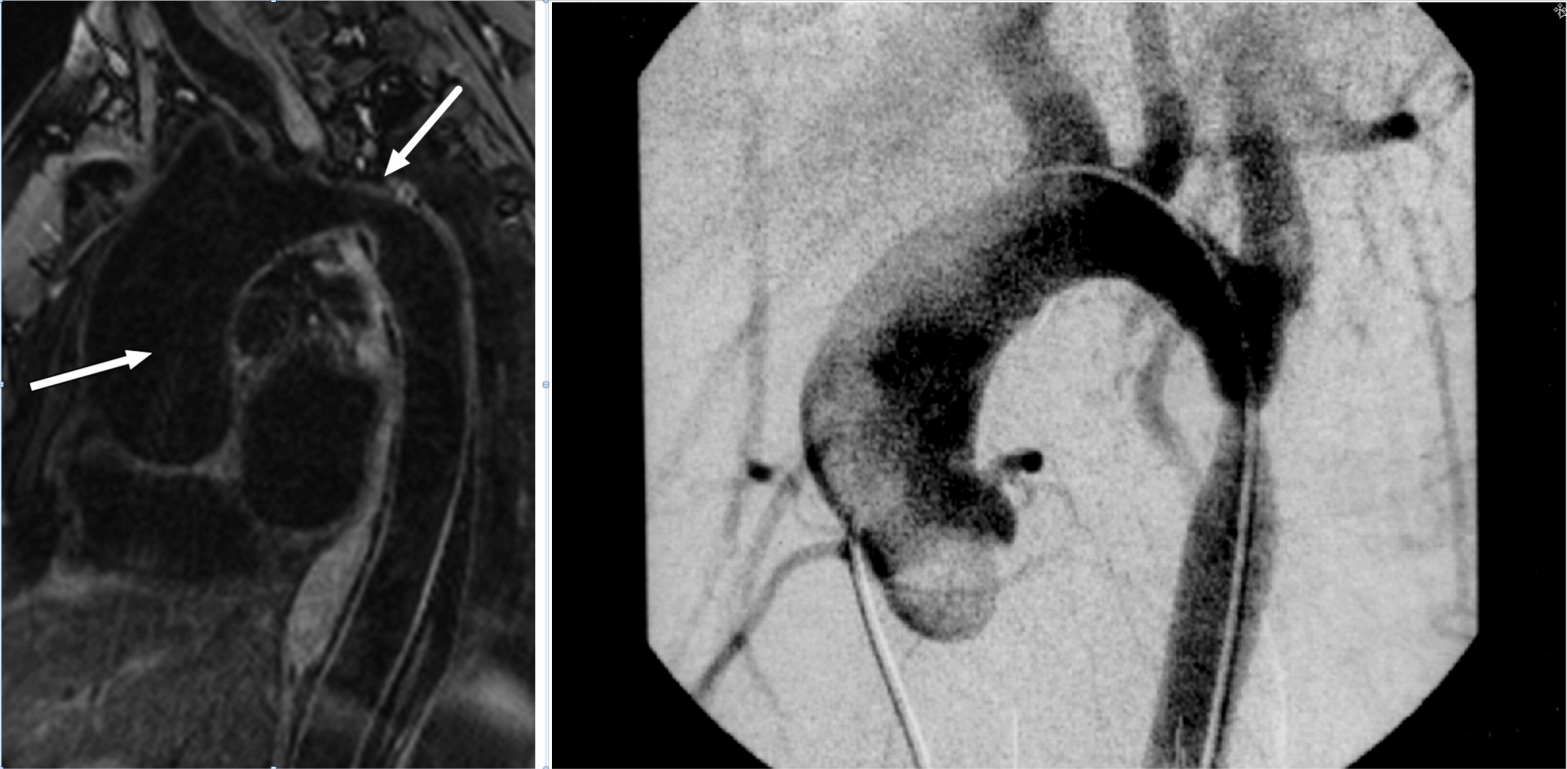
MRI of the chest, depicting the ascending thoracic aneurysm leading to a tight coarctation of the descending aorta. The Aortogram also delineates similar pathology.
